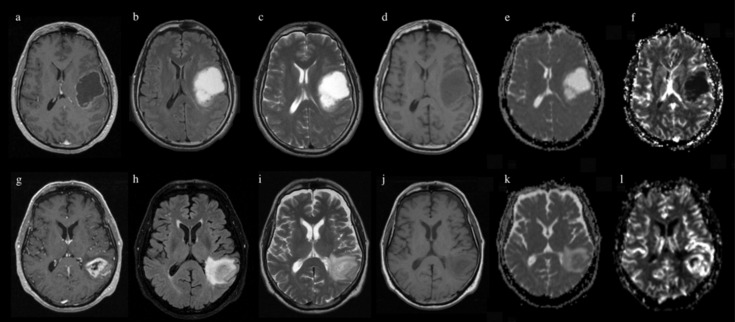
Examples of correctly predicted outcome (IDH mutation) from our CNN model. Images above show MPRAGE (a), FLAIR (b), T2 (c), T1 (d), ADC (e) and rCBV (f) sequences of a patient with IDH mutated GBM. Images below show MPRAGE (g), FLAIR (h), T2 (i), T1 (j) ADC (k) and rCBV (l) sequences of a patient with IDH-wild-type GBM.
